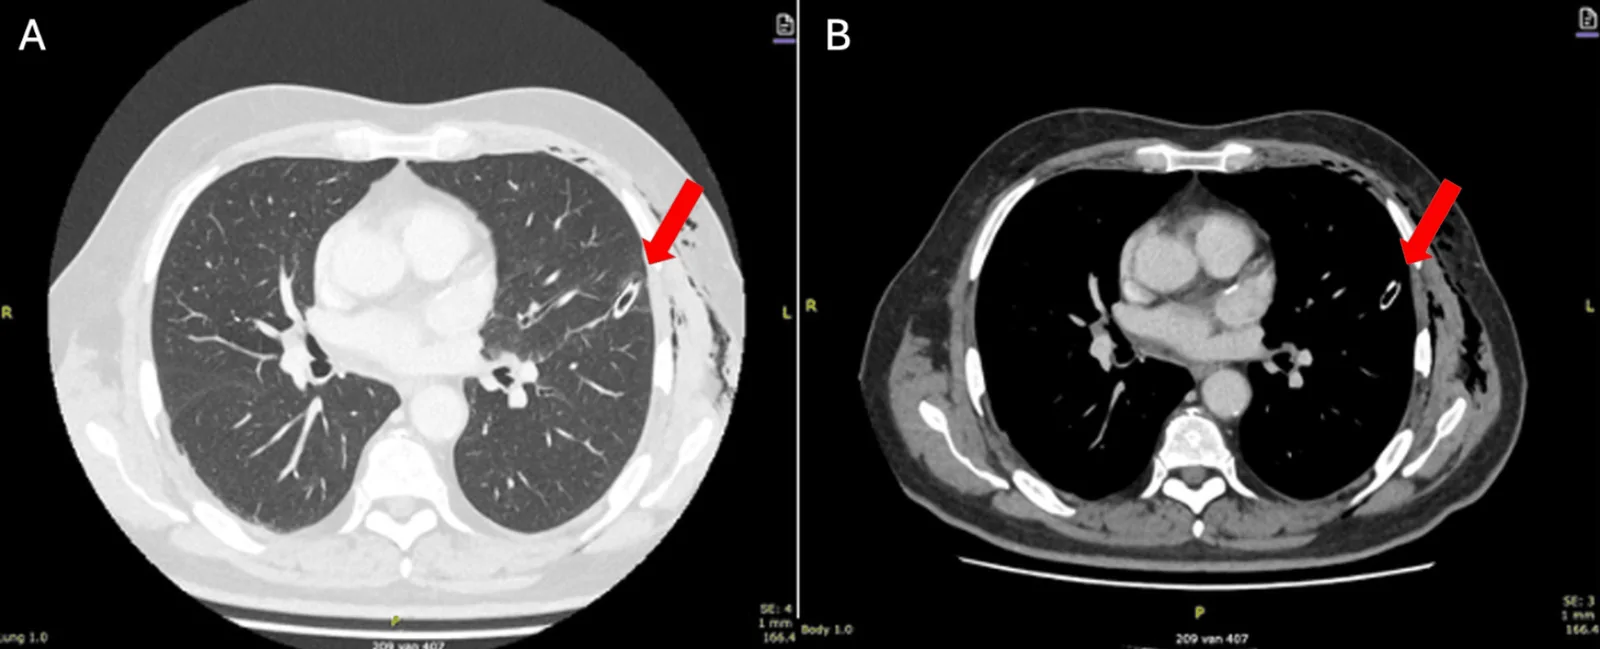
CT imaging with chest drain in situ and no signs of pleural abnormalities. (A) Lung-window images with the chest drain in situ, indicated by the red arrow. (B) Mediastinal-window images with the chest drain in situ, indicated by the red arrow. CT: computed tomography
